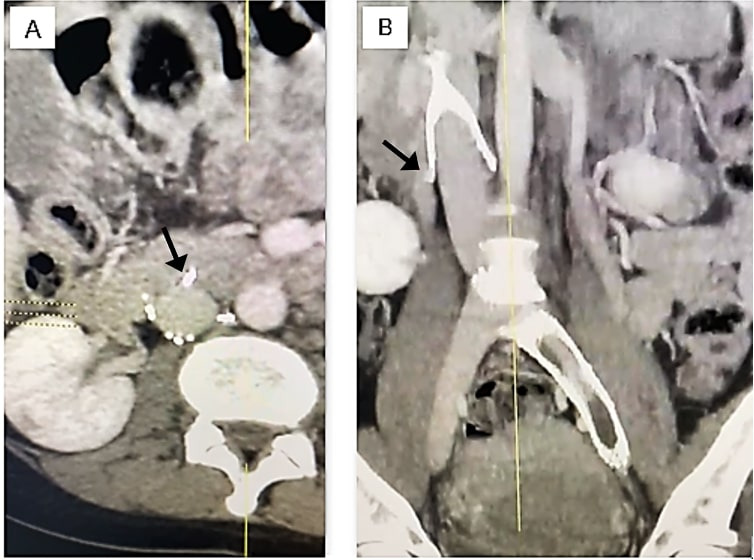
Vena cava filter perforating the inferior vena cava. (A) Axial computed tomography; (B) Coronal computed tomography. Arrowhead: filter securing struts perforating the wall of the vena cava. Source: Authors’ personal archives.
